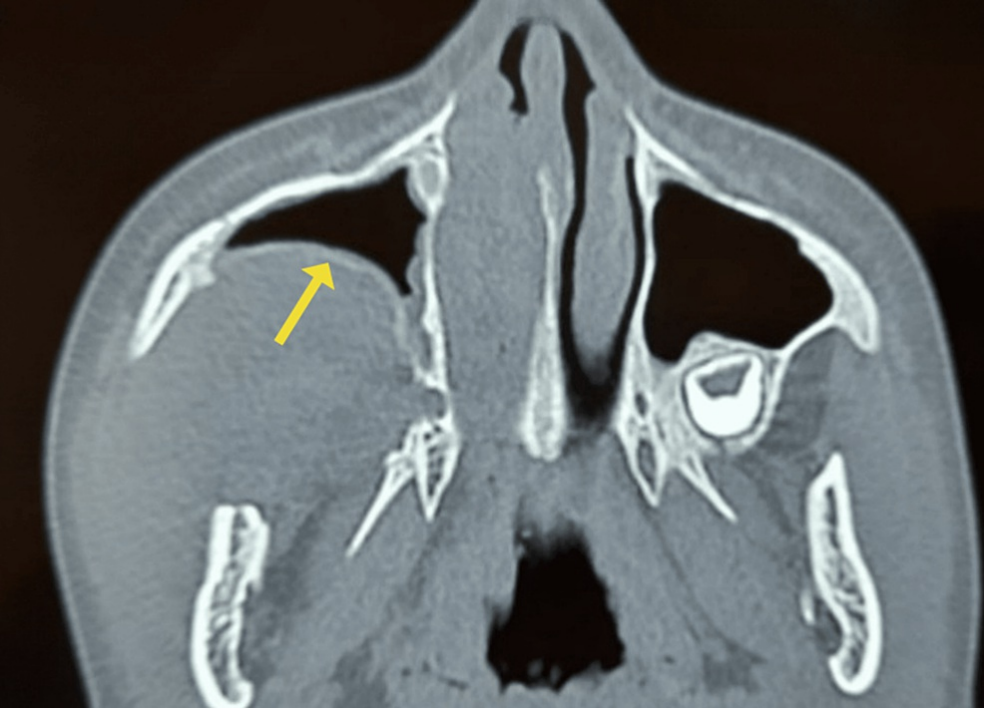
Well-defined soft density mass lesion causing anterior bowing of posterior wall of the maxillary sinus as seen in computed tomography (axial view)
